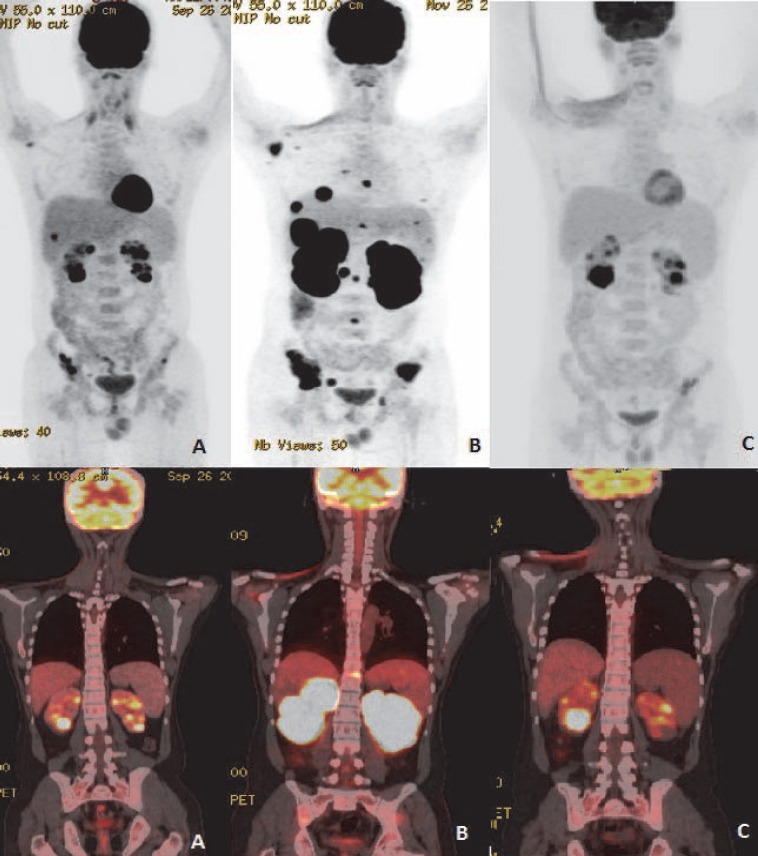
3D MIP and reformatted coronal fused PET/CT images of NHL involving kidney: (A) Multifocal FDG avid cortical lesions involving both kidneys. (B) PET/CT scan for response assessment post 3 cycles chemotherapy shows marked metabolic progression with diffusely increased parenchymal FDG avidity of both kidneys. (C) PET/CT scan done after completion of second line chemotherapy shows partial metabolic response
